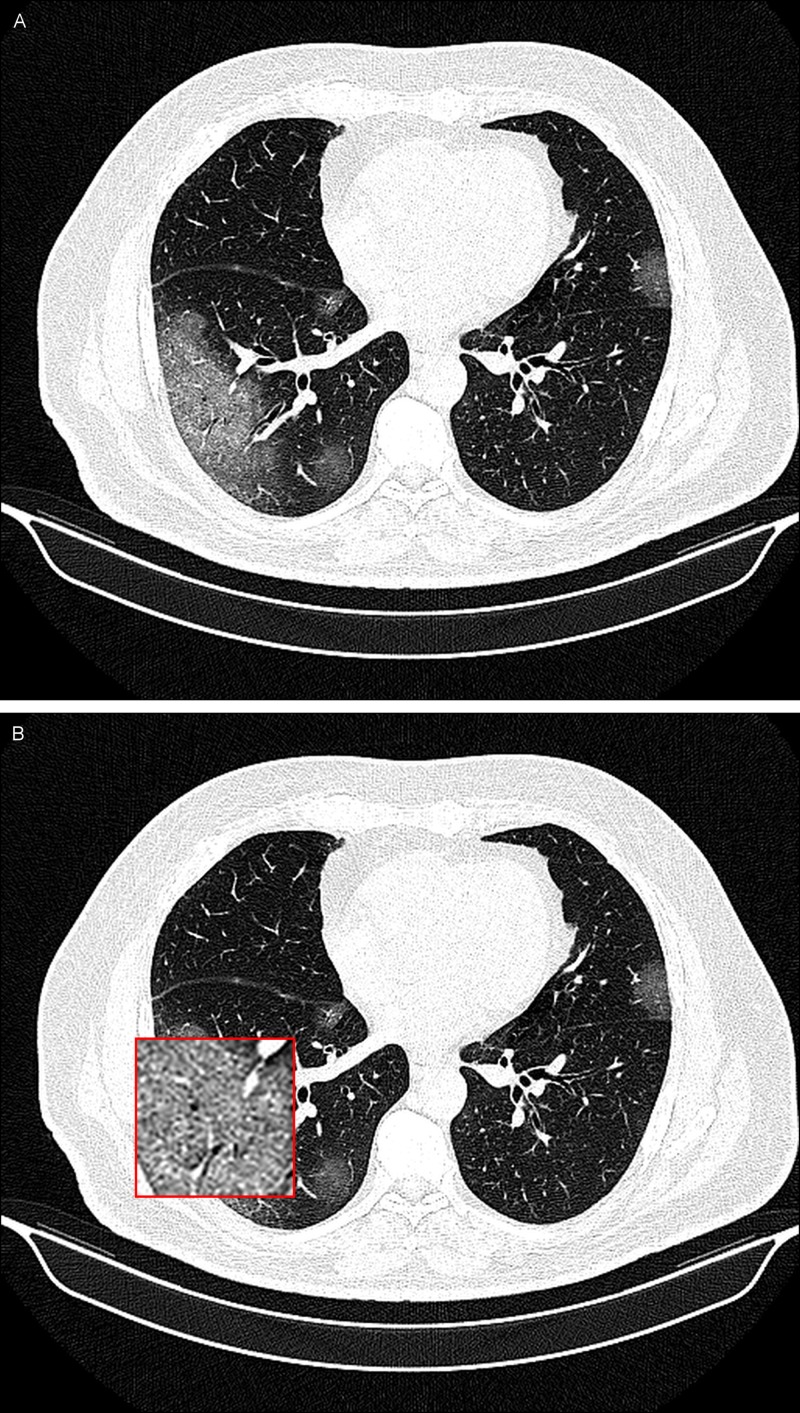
A 45-year-old woman with COVID-19 infection. A noncontrast-enhanced CT image of the lungs demonstrating bilateral patchy ill-defined ground-glass opacities (GGOs) in posterior segments of the lungs (A) and an inset magnified view of the lesion for better delineation at the location of GGO in the right lower lobe of the lung (B).
